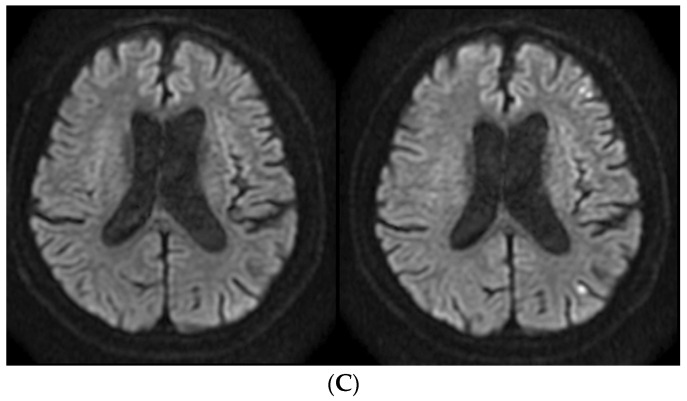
(A) Preoperative brain perfusion single-photon emission computed tomography in a 74-year-old man with symptomatic left internal carotid artery stenosis (90%) shows reduced cerebral blood flow (left) and reduced cerebrovascular reactivity to acetazolamide (center) in the left cerebral hemisphere where hyperperfusion develops immediately after surgery (right); (B) Transcranial Doppler recording during exposure of the carotid arteries in the patient of Figure 3A shows three microembolic signals (arrows) in the power spectrum display of left middle cerebral artery blood flow. This patient had a total of 10 microembolic signals during exposure of the carotid arteries; (C) A diffusion-weighted image 6 h after surgery in the patient of Figure 3A,B shows development of new postoperative multiple high-intensity lesions in the left cerebral hemisphere (right) when compared with a preoperative image (left). These lesions did not change on diffusion-weighted imaging 24 h after surgery. This patient suffered slight motor weakness in the right upper extremity after recovery from general anesthesia, and this deficit resolved completely within 12 h.
